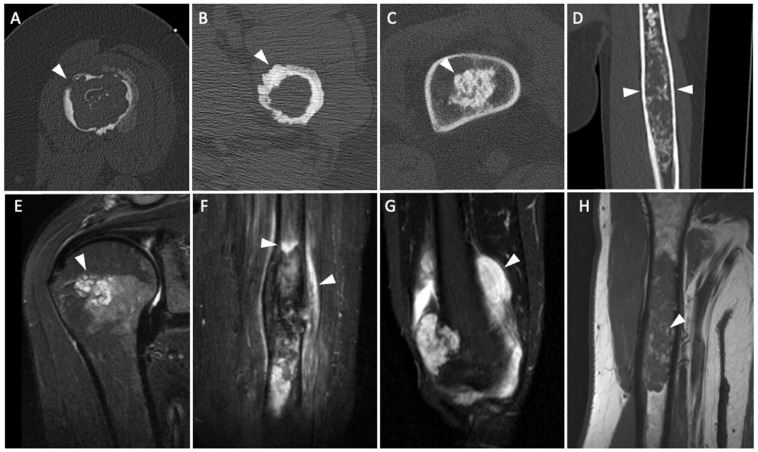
Exemplary computed tomography images demonstrating the features endosteal scalloping, cortical penetration (A), periosteal reaction (B), Calcifications >2/3 (C) and Expansion of the bones (D) as well as magnetic resonance (MR) images for discontinuous growth pattern (short-tau inversion recovery (STIR) image) (E), edema within the bone and adjacent soft tissue (short-tau inversion recovery (STIR) image) (F), extraosseous soft tissue component (short-tau inversion recovery (STIR) image) (G) and fat entrapment (T1 weighted image) (H). Features are highlighted by white arrow heads.
